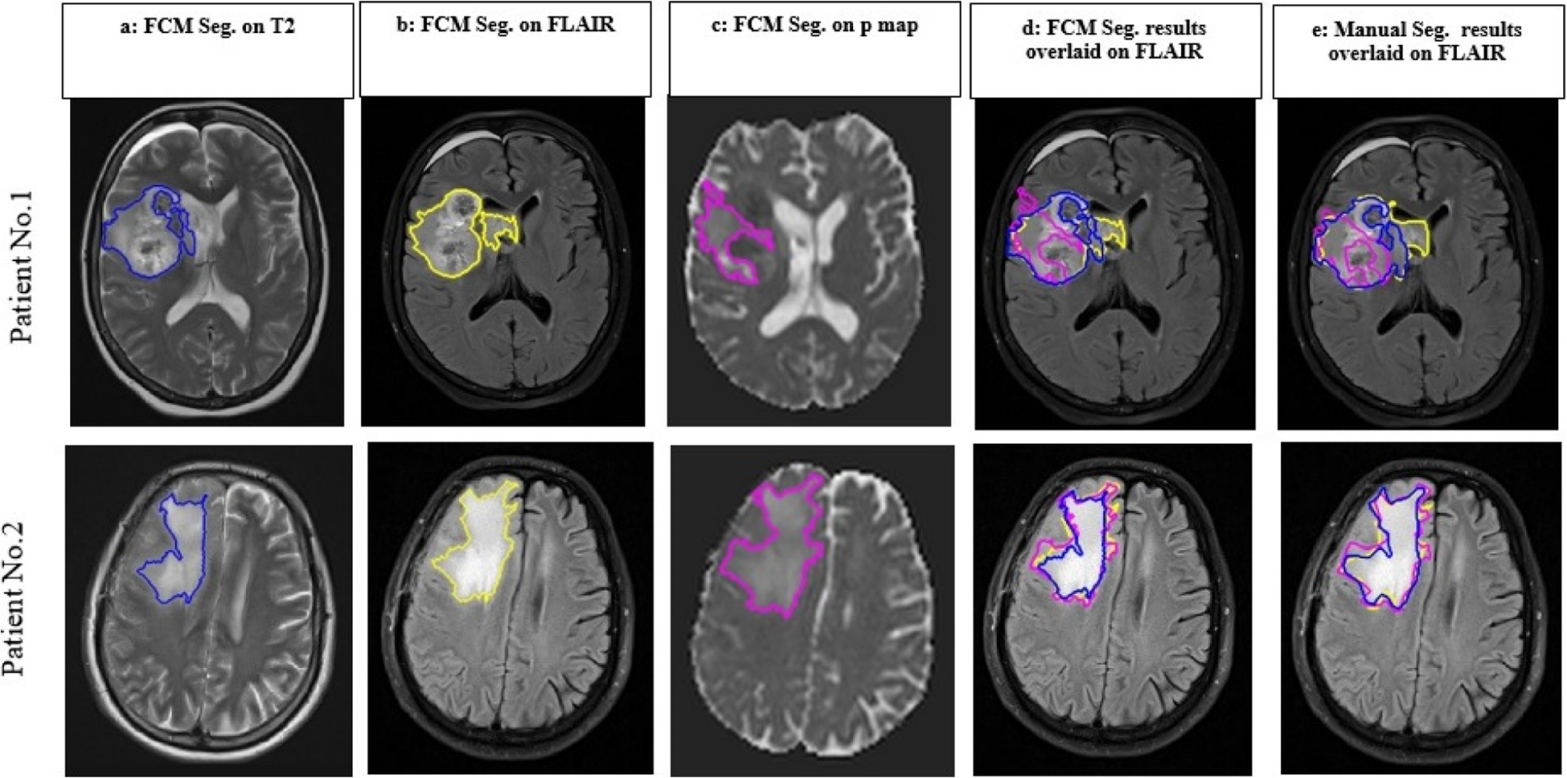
Results of segmentation on two patient’s data with glioblastoma who had undergone partial resection. Columns (a-c): FCM segmentation on (a) *T*_2_ (blue), (b) *T*_2_*-FLAIR* (yellow), (c) *p* maps (pink). (d) results of FCM segmentation overlaid on *T*_2_*-FLAIR* images, (e) Manual segmented region on three images overlaid on *T*_2_*-FLAIR*. First row represents the less agreement in location between *T*_2_*/T*_2_*-FLAIR* and *p*-map (DI_TF_ = 0.26, DI_TP_ = 0.57_,_
